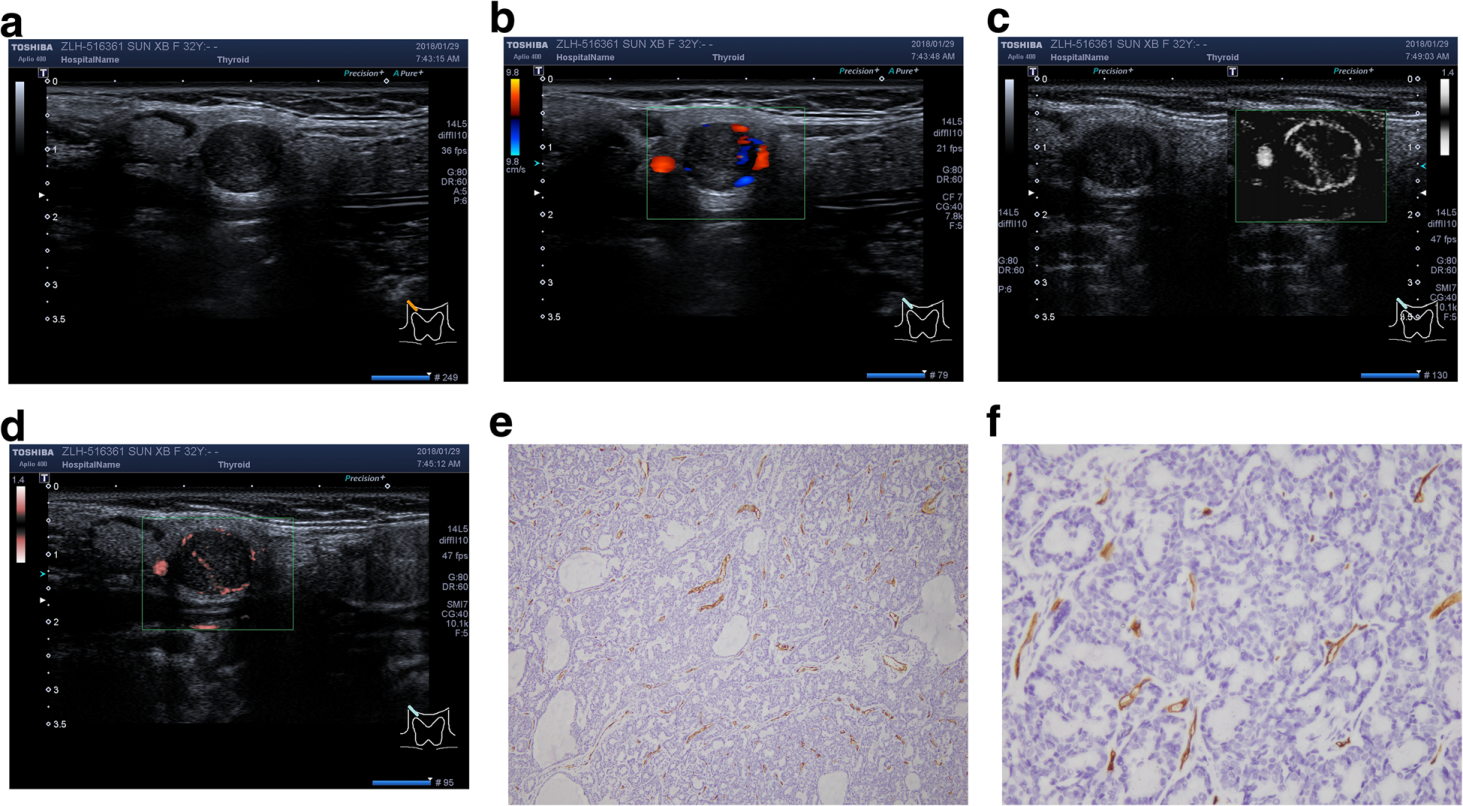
Represent BCA case from left parotid gland. a. Conventional ultrasound image; b. CDFI: punctate or fine rod vessels, Mixed distribution, Alder 3; c. mSMI and d. cSMI: dense streak blood stream, Mixed distribution, Alder 3. VIby cSMI:18.1; e. MVD by IHC (× 100); f. MVD by IHC: 62 (× 400)
